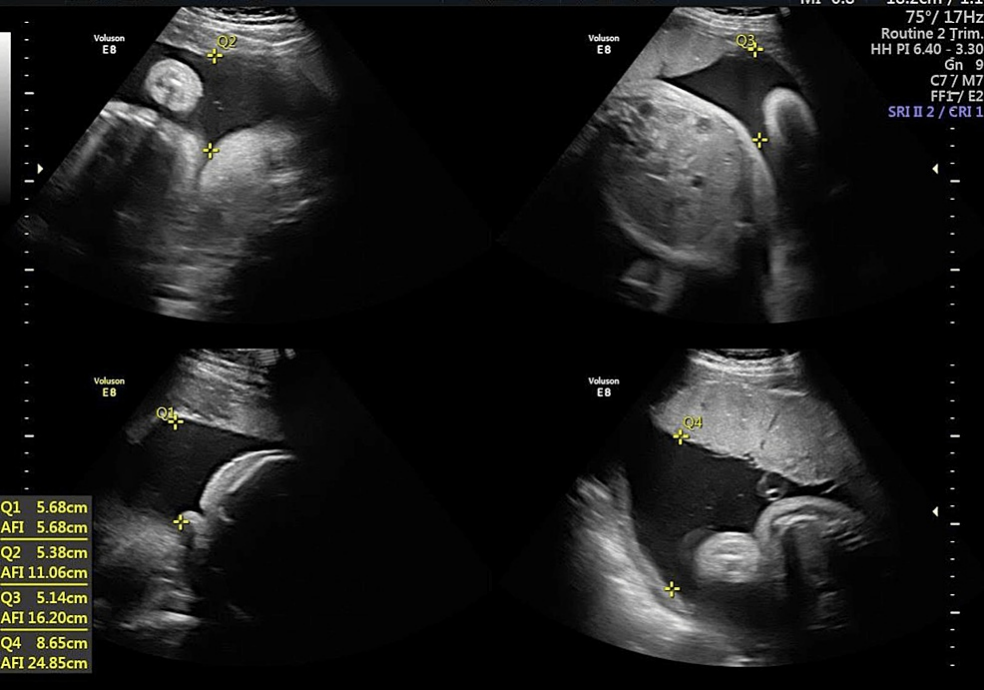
Ultrasound image at 31 weeks of gestation showing polyhydramnios.
